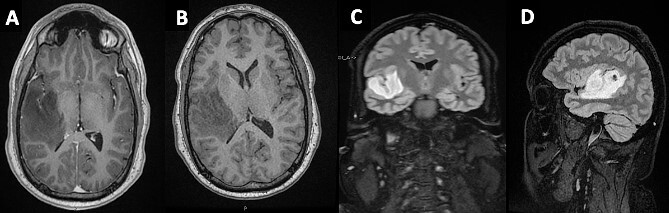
Mass lesion occupying the HG on the right temporal gyrus on (A) axial post-contrast T1 - (B) axial non-contrast T1- (C + D) T1-weighted sagittal and coronal FLAIR MRIs, respectively.
